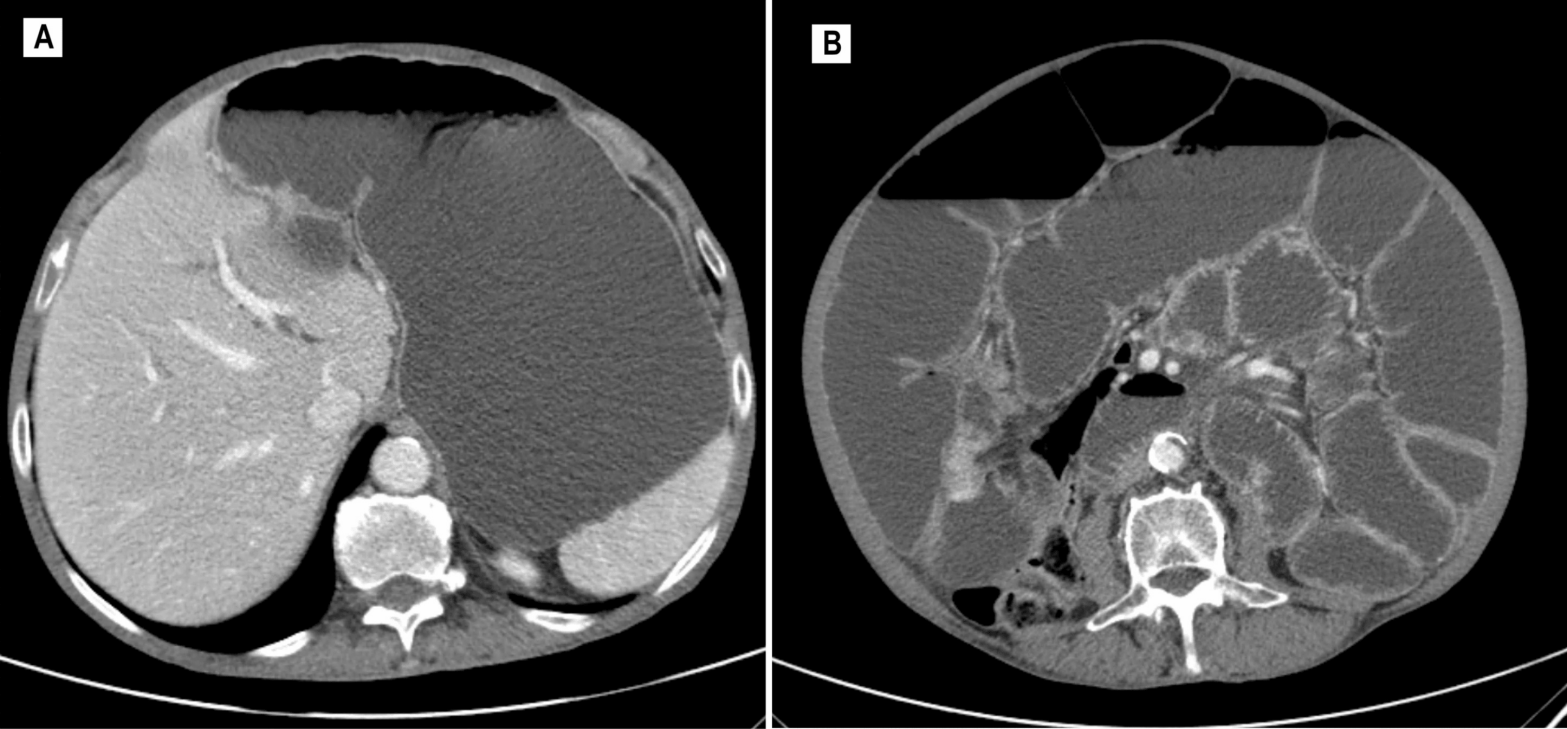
Abdominal and pelvic CT scan displaying notable gastric distention (A) and small-bowel distension (maximum caliber: 74 mm), leading to large bowel collapse (B). CT: Computed tomography
